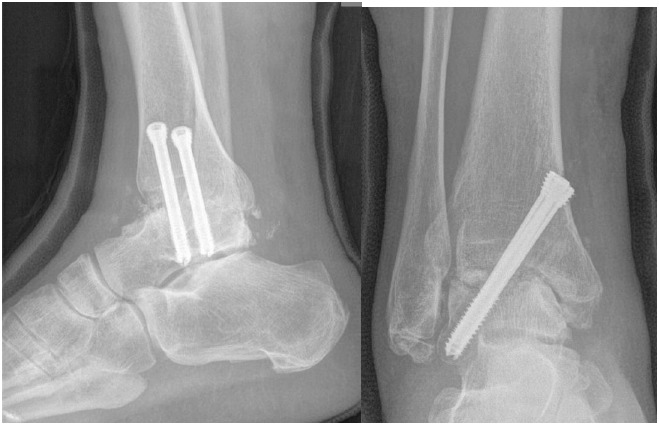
Non-weight bearing AP and lateral radiographs two weeks after AAA with two parallel HCS compression. Note distal HCS threads are within lateral talar process and proximal covered in thinner medial tibial cortex causing any impingement on soft tissues.
